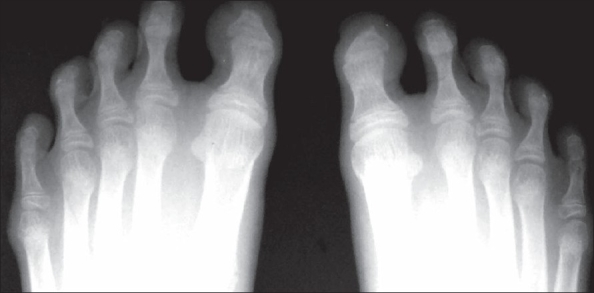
Radiograph of both feet shows acro-osteolysis
